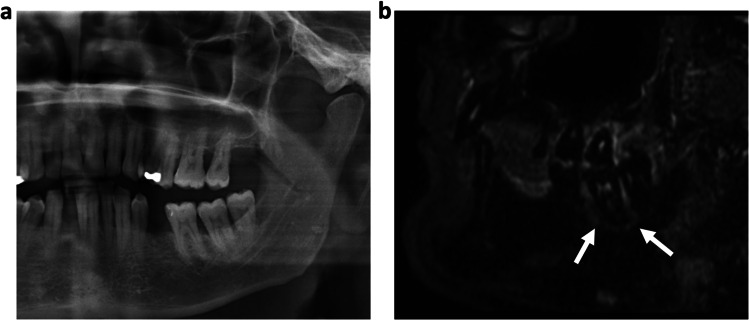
a OPT of a non-symptomatic 58-year-old patient. There are no periapical radiolucencies detected on conventional OPT. In contrast, the sagittal reconstruction of the 3D STIR sequence (b) shows a bright periapical bone marrow edema around the root of the tooth 37, indicating the formation of an inflammatory complex that has contact to the adjacent roots (white arrows). The missing radiolucency in the OPT may indicate that it is an early-stage inflammation without osteolysis of the alveolar bone
